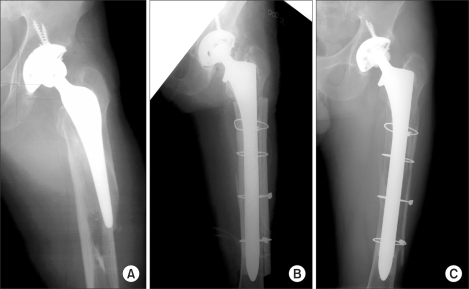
Radiographs show a 59 year-old man with periprosthetic fracture. (A) Preoperative radiograph reveals a periprosthetic fracture with Paprosky type III B femoral bone defect. (B) Revision total hip arthroplasty was performed with solution bowed stem and cortical strut allo-bone. (C) Five years after revision total hip arthroplasty, grafted cortical strut allo-bone had been incorporated .
